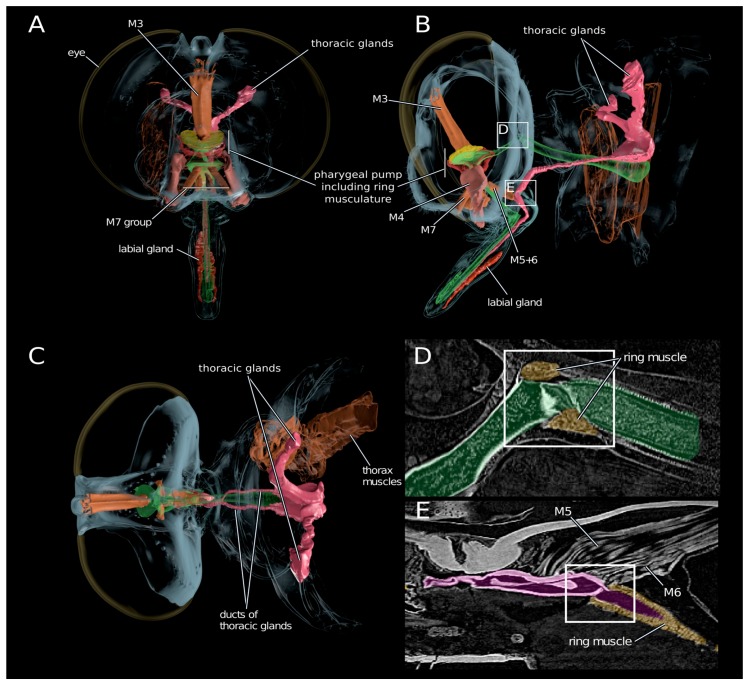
3D reconstruction of the gland and head morphology in *E. rufibarbis.* Reconstruction based on synchrotron micro-computed tomography data. (A) Frontal view; (B) Lateral view; (C) Dorsal view; (D,E) Images of the original scan data showing the digestive tract with the ring musculature to close the pharyngeal tract (D) and the musculature attaching at the salivary pump system (E). Note the “closed” position of the valve at the center of the white frame.
